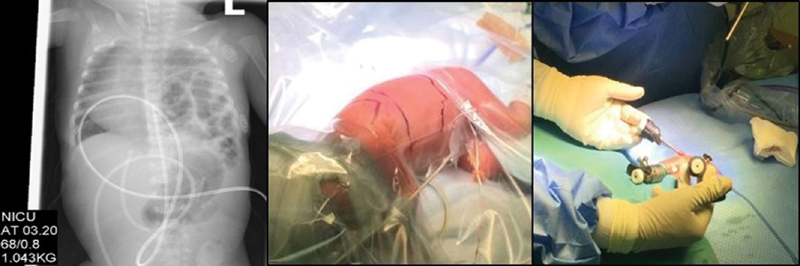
Postnatal X-ray, left-sided congenital diaphragmatic hernia. Patient position and placement of the ports. NICU, neonatal intensive care unit.
